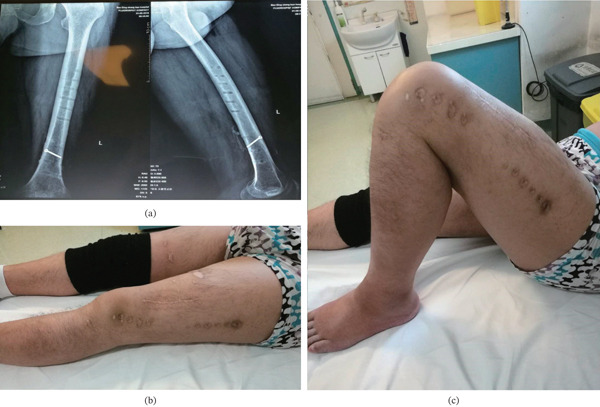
(a) Anteroposterior (left) and lateral (right) radiographs of the left femur obtained at the 20‐week postoperative follow‐up, demonstrating solid bony union with complete disappearance of the fracture line. The modified RUST score was 16, confirming bridging across all four cortices. (b, c) The surgical incision was well‐healed, and the range of motion at the knee was extension 0° to flexion 130°.
